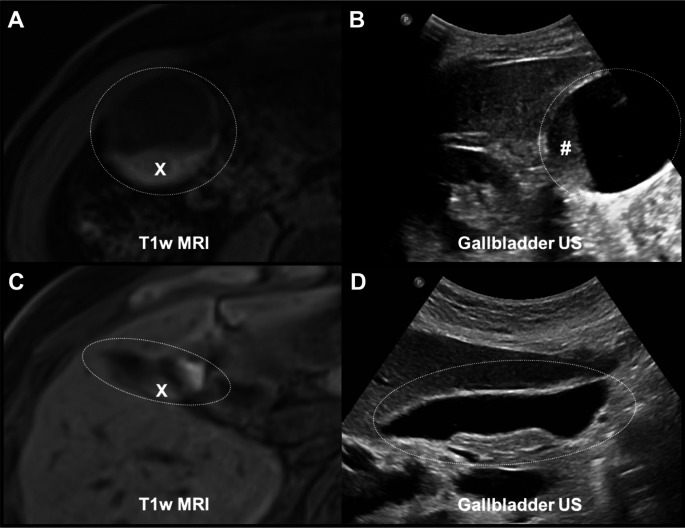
Examples of MRI findings in patients with confirmed and unconfirmed gallbladder sludge. Panel A: T1-weighted (T1w) MRI showing pronounced hyperintensity layering (x), confirmed as sludge (#) by ultrasound (US) in Panel B. Panel C: T1w MRI with light hyperintensity layering (x), suggestive of sludge but not confirmed by the US in Panel D. The gallbladder is delineated with a dotted line
